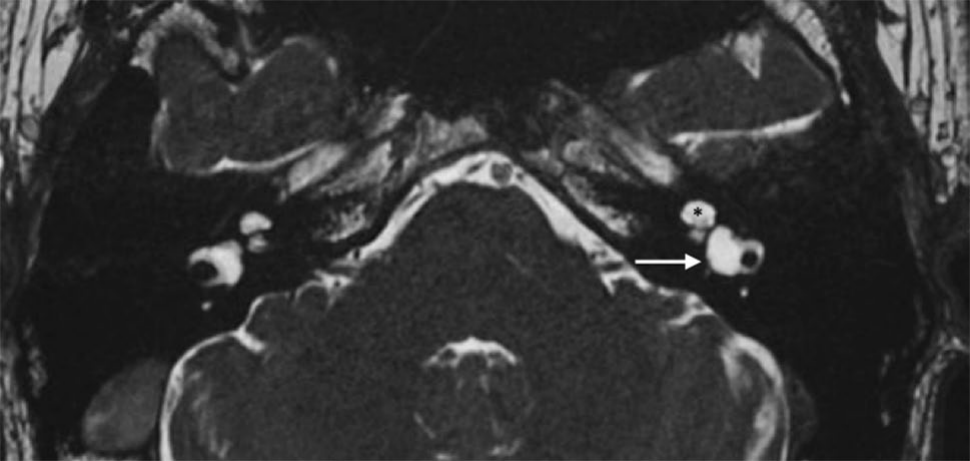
Incomplete partition type I. A 5-year-old female with profound right SNHL. A 45-year-old-man with progressive left HL. Axial MRI T2 high-resolution 3D sequence obtained at IAC level shows typical IP-I malformation abnormalities on the left side (arrow) with a cystic appearance of the cochlea (asterisk) that is clearly separated from the vestibule
